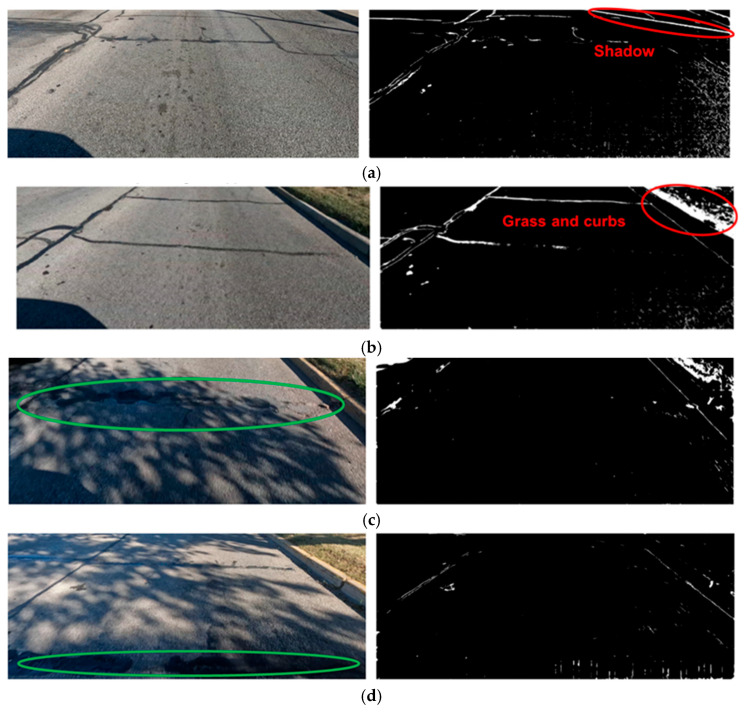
CT-CrackSeg detection errors: false positives from (a) sign shadows and (b) grass/curb regions, and false negatives for (c,d) surface deformations.
